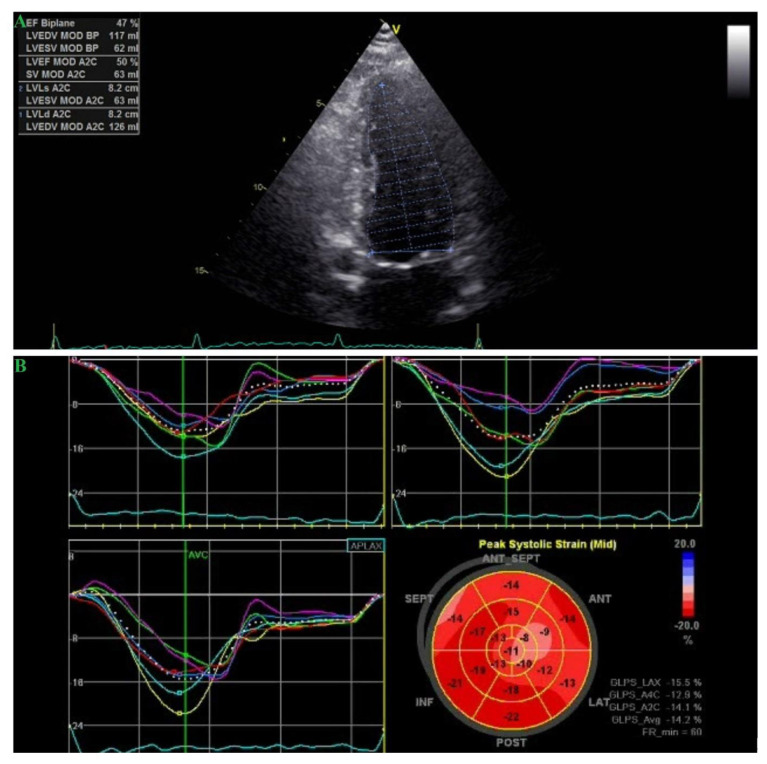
Transthoracic echocardiography: wall motion abnormalities in the left anterior descending artery territory with hypokinesia of the apical segments of the anterior and lateral wall and of the apex; borderline left ventricular systolic dysfunction (A) (EF(Ejection fraction) Biplane = 47%); (B) reduced peak systolic strain (Mid)—global longitudinal peak strain average (GLPS_Avg) 14.2%.
